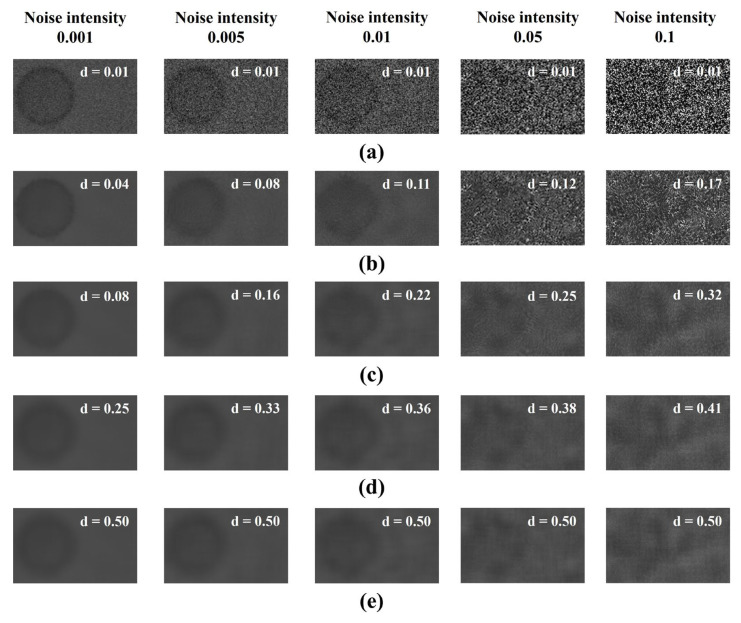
The results are magnified regions of Figure 4, using the fast non-local means (FNLM) noise reduction algorithm with various smoothing factors (*d*) on a computed tomography (CT) image with Gaussian noise added with a standard deviation: (a) low smoothing factor, (b) semi-low smoothing factor, (c) optimized smoothing factor, (d) semi-high smoothing factor, and (e) high smoothing factor.
